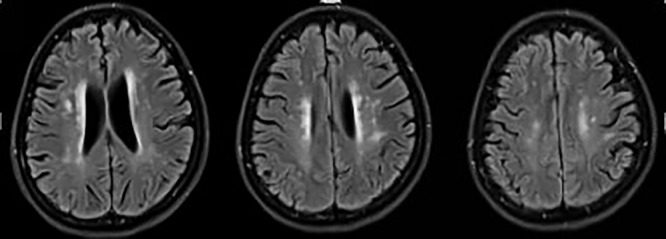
Head MRI and T2WI images of III9 (currently no clinical symptoms). Image shows bilateral lateral ventricles, bilateral semi-oval center with multiple patchy abnormal signal, and the white matter of the bilateral lateral ventricle slightly changed.
